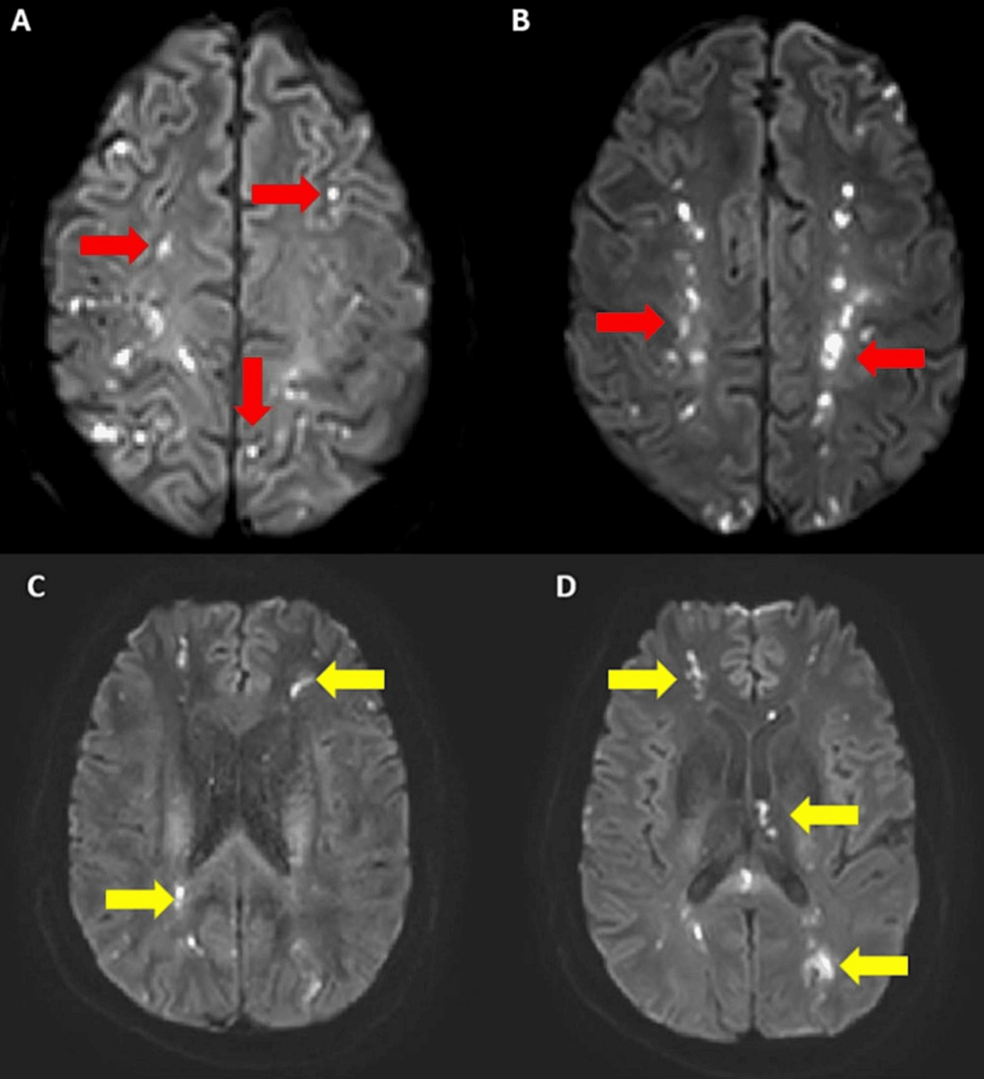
A-B: DWI sequence showing multiple cortical acute infarcts in bilateral hemispheres (red arrows). C-D: sequence showing multiple subcortical acute infarcts in bilateral hemispheres (yellow arrows). Diffusion-weighted imaging
